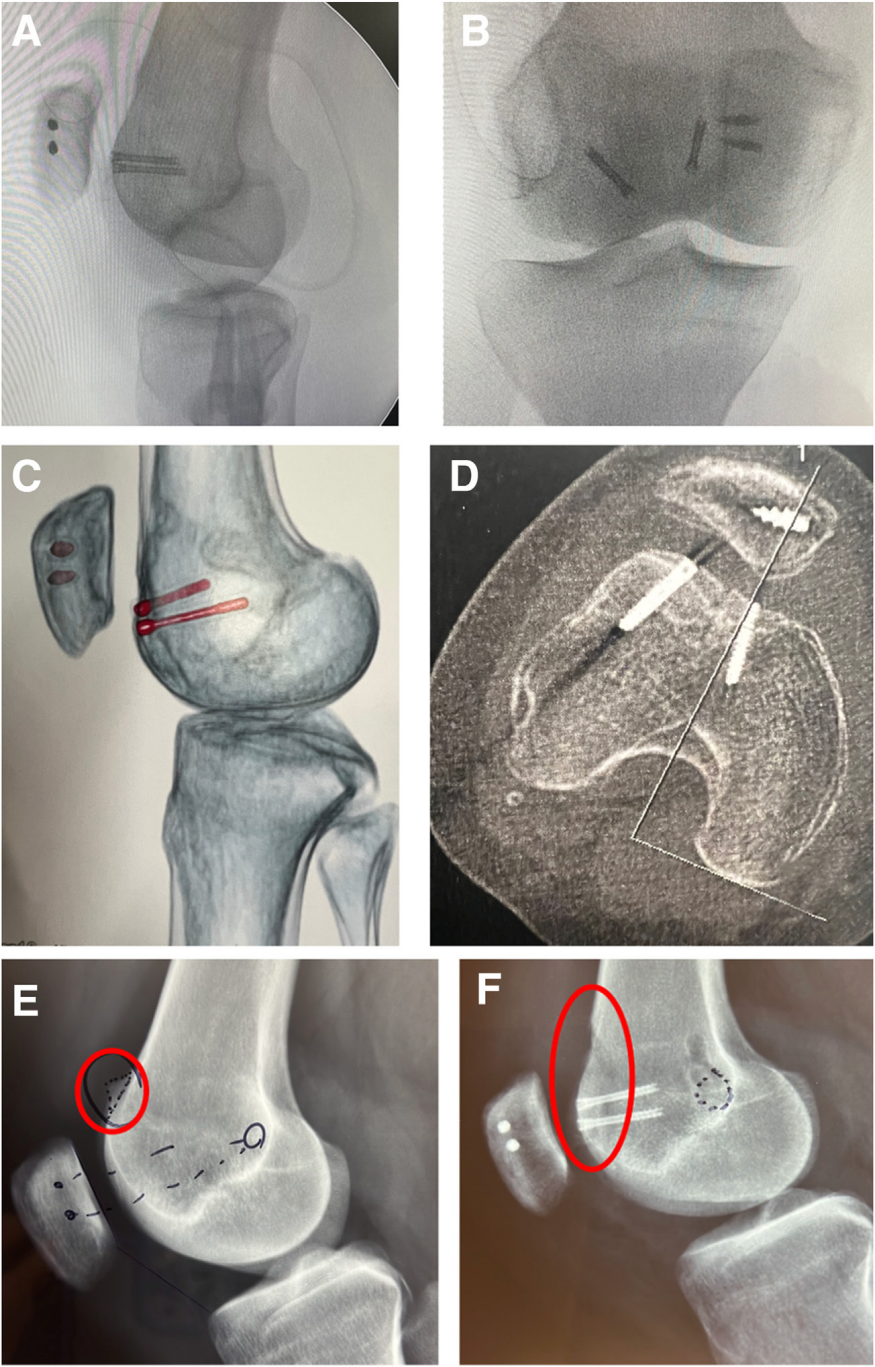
Imaging of modified trochleoplasty. (A, B) Postoperative control fluoroscopy. (C, D) Postoperative computed tomography scan. (E, F) Preoperative and postoperative radiographs (right knee). Oval indicates preoperative trochlear proeminence (E). Oval indicates postoperative trochlear proeminence (F).
